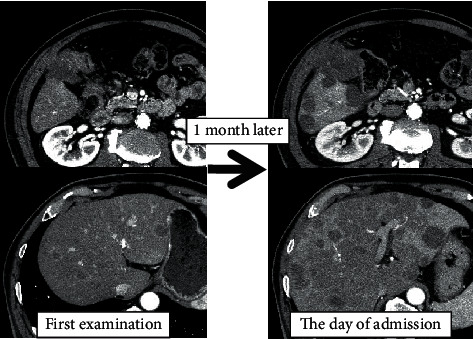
Contrast-enhanced CT on admission shows rapid progression of the primary tumor and liver metastases compared with that in the initial examination 1 month prior.
